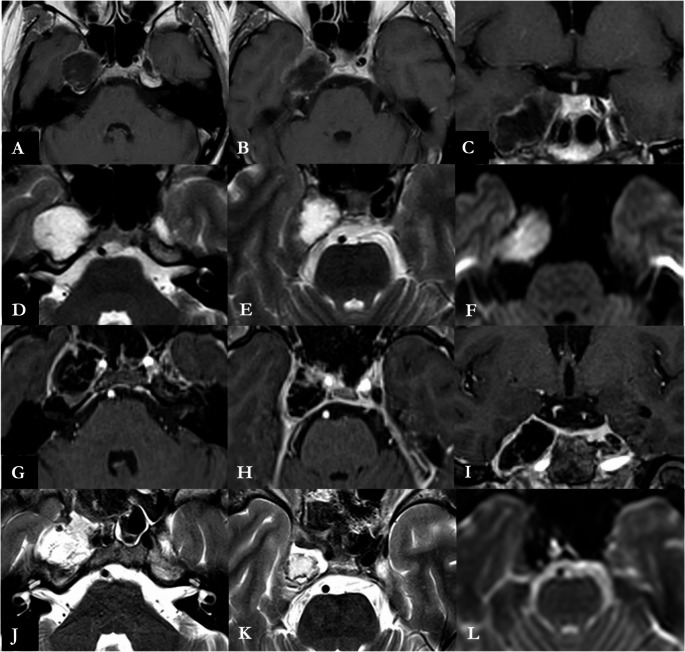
Preoperative axial (A, B) and coronal (C) contrast enhanced T1 – weighted, axial (D, E) T2 – weighted and axial (F) DWI MRI images showing a non – enhancing diffusion – restricting lesion of right Meckel’s cave. The postoperative axial (G, H) and coronal (I) contrast enhanced T1 – weighted, axial (J, K) T2 – weighted and axial (L) DWI MRI images reveal a gross – total resection of cystic componentPreoperative axial (A, B) and coronal (C) contrast enhanced T1 – weighted, axial (D, E) T2 – weighted and axial (F) DWI MRI images showing a non – enhancing diffusion – restricting lesion of right Meckel’s cave. The postoperative axial (G, H) and coronal (I) contrast enhanced T1 – weighted, axial (J, K) T2 – weighted and axial (L) DWI MRI images reveal a gross – total resection of cystic component
